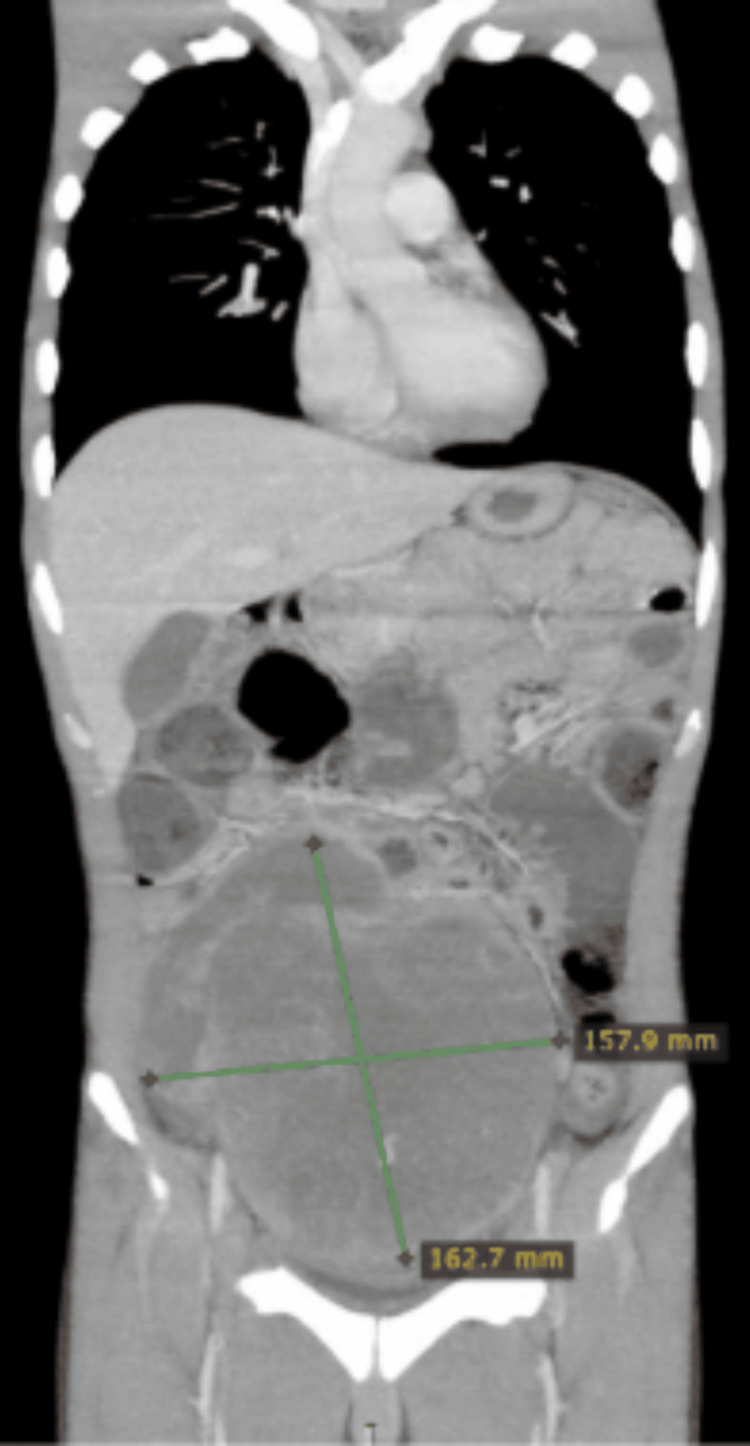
Coronal plane of the CT scan showing a mass of around 11×15 cm CT: computed tomography
